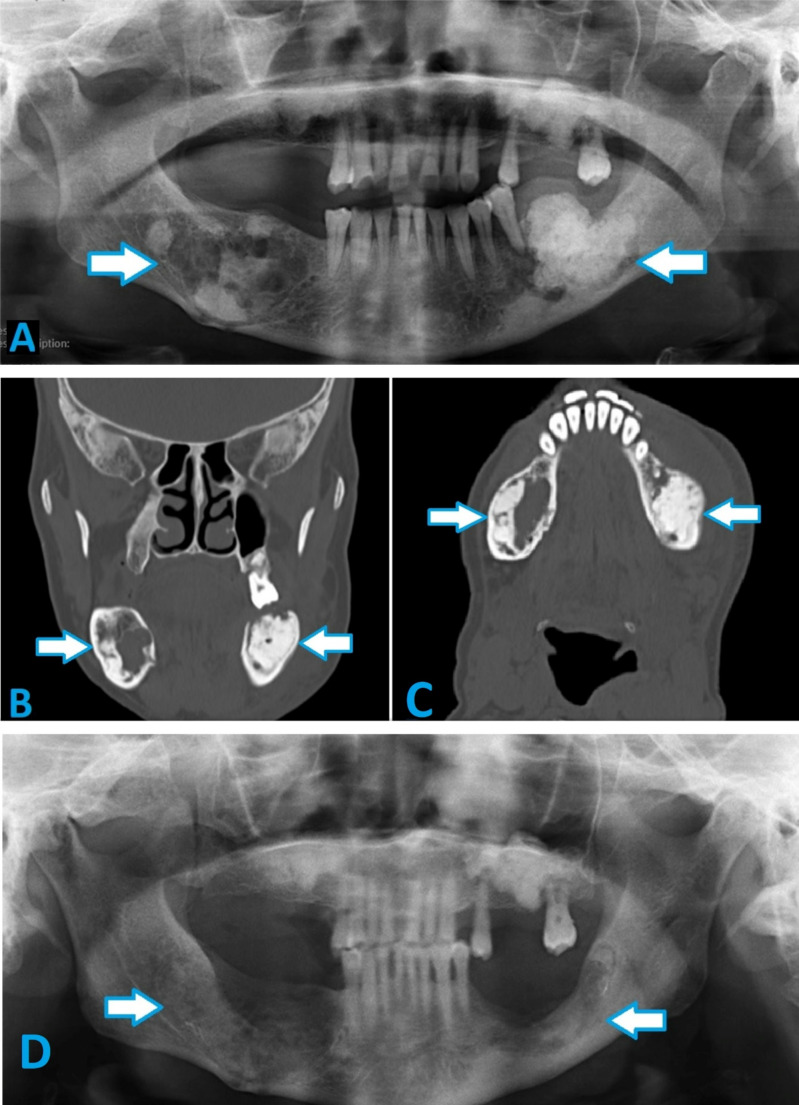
(a) Pre-operative panoramic radiograph of a patient diagnosed with florid COD complaining of pain and bilateral swelling in the mandible. (b) Pre-operative coronal and (c) axial CBCT images revealing expansive mixed density lesions in the bilateral mandible. (d) Seven months post-operative panoramic radiograph of the same patient showing good healing following excision of COD lesions from the posterior mandible
